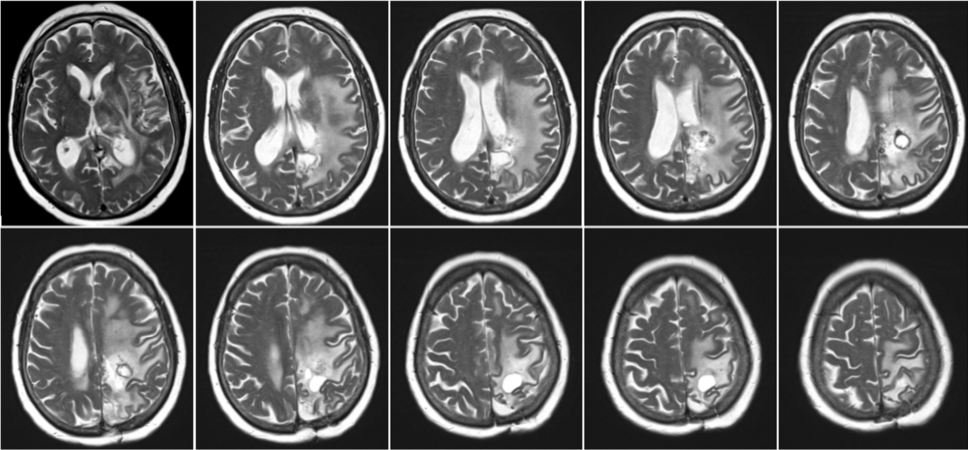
T2-weighted MRI one year after initial presentation and 10 months after left mesioparietal resection. Increase in size of the left posterior cyst as well as periventricular intracystic bleeding. Stable course of the leukoencephalopathy
